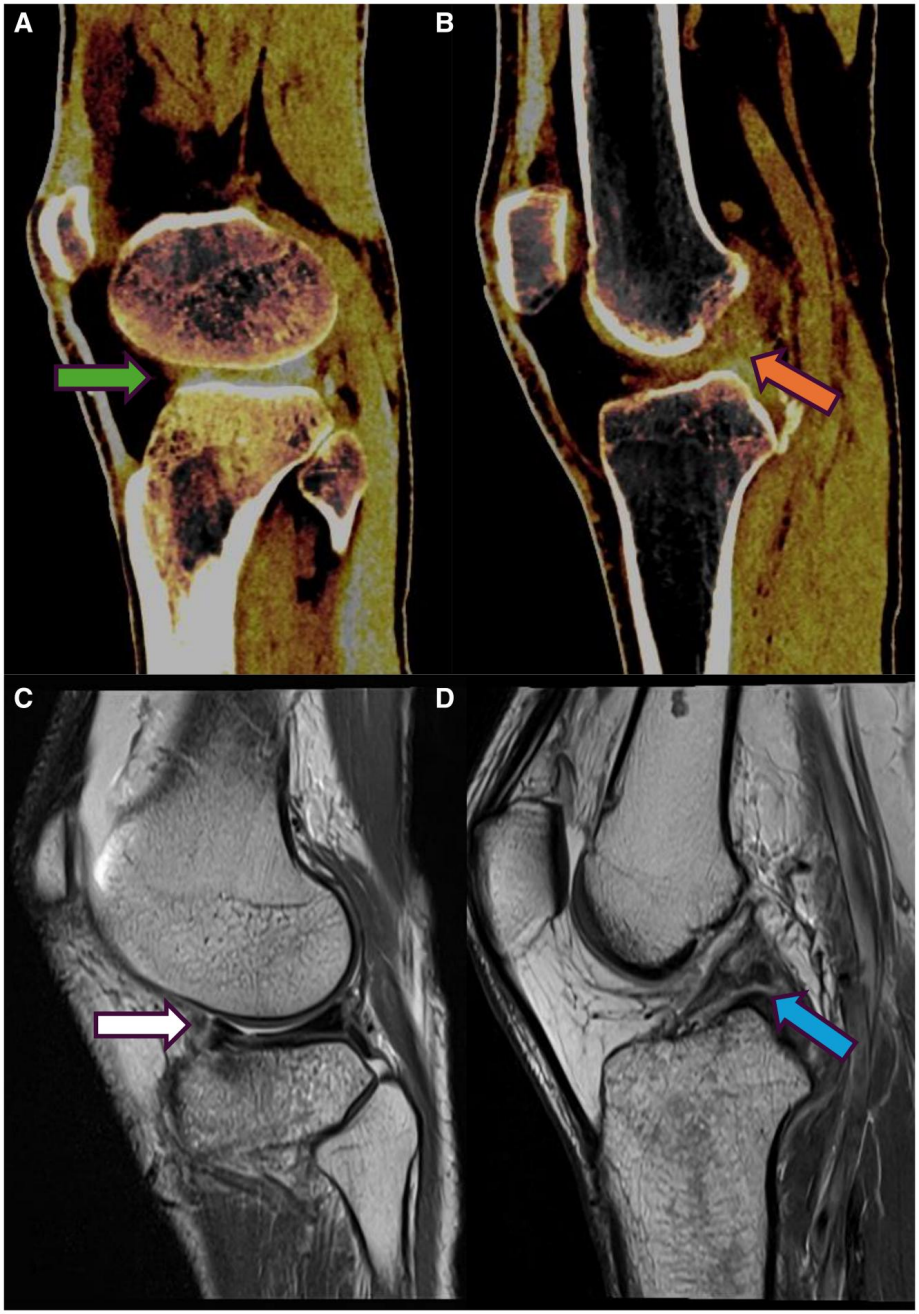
Patient with a history of acute trauma. A (green arrow): injury of the anterior horn and B shows injury of ACL as depicted on the DECT tendon map application. Findings were confirmed with MRI as shown in C and D.
